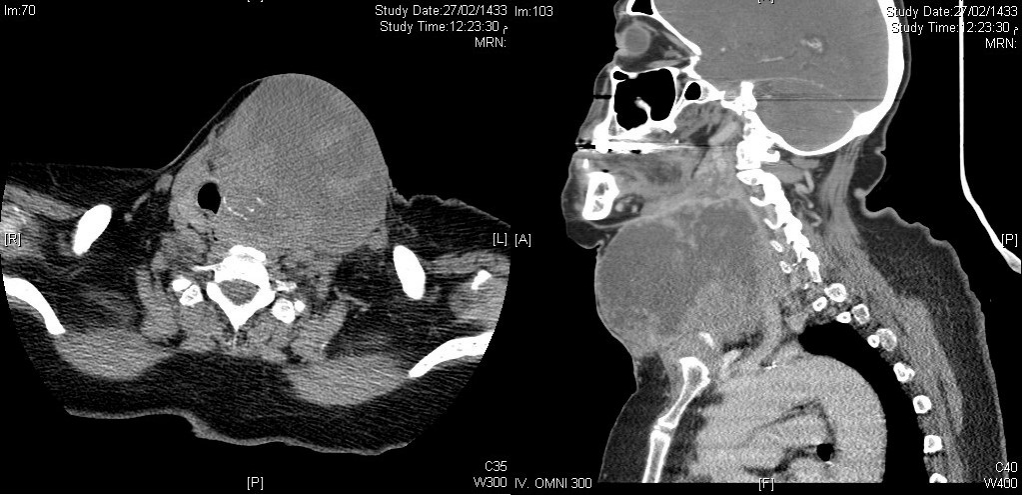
Computed tomography (CT) neck showing 10 × 10 cm mass in left lobe of thyroid, partially necrotic invading to adjacent skin and trachea and no cervical lymphadenopathy.
